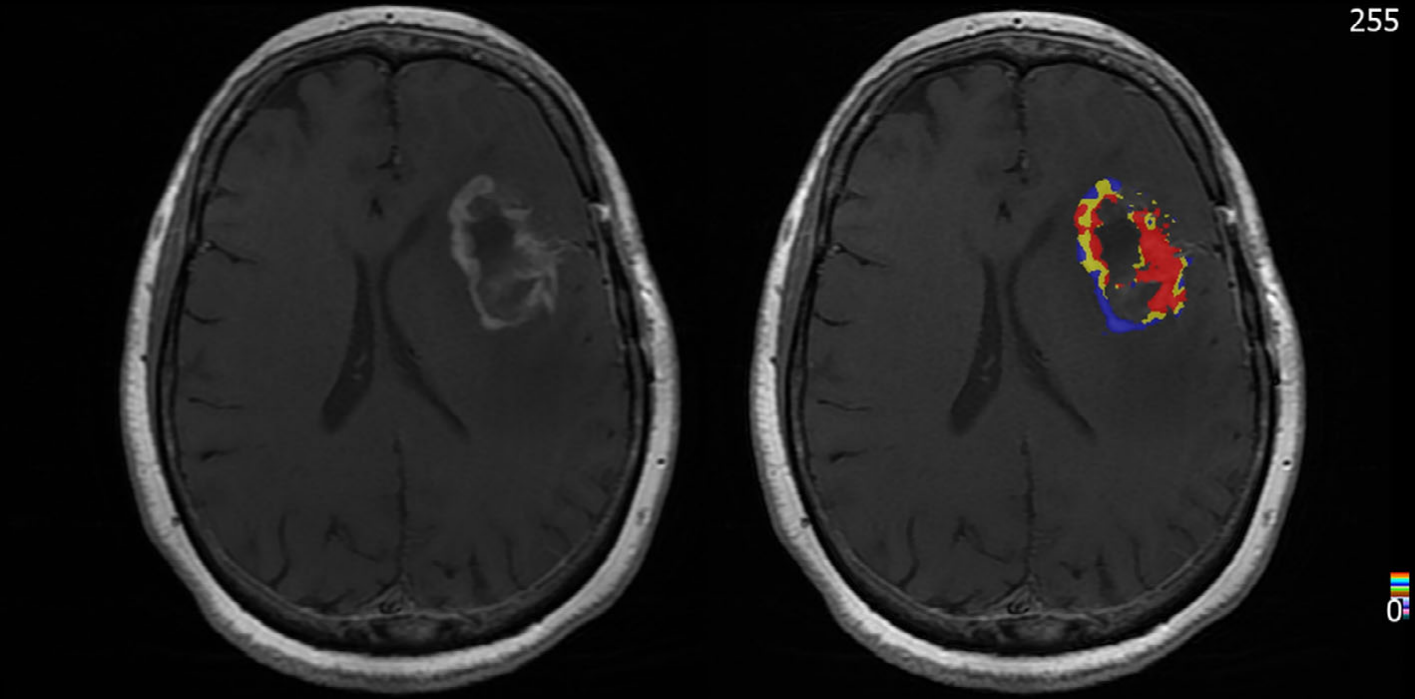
Patient with recurrent glioblastoma. (a) T1w MRI with CEA, (b) corresponding map of fractional tumor burden (FTB) showing regions of zero‐low (blue), intermediate (yellow), and high rCBV (red)within the contrast agent enhancing region.
